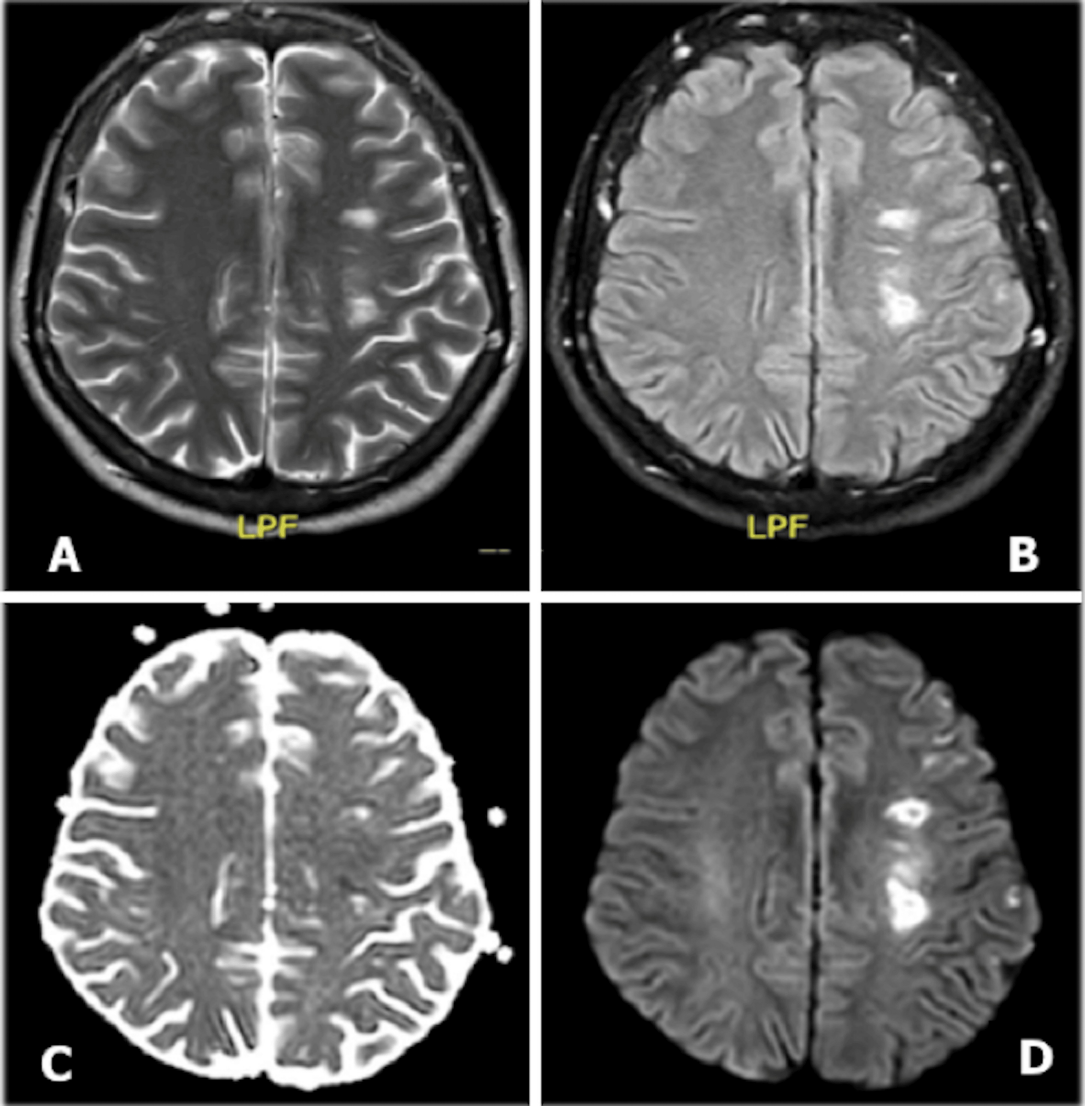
Magnetic resonance imaging with contrast of the brain. Ischemic infarcts in the middle cerebral artery territories, in different stages (acute, subacute, and chronic, with alteration of flow signal in the left carotid artery. (A) T2 sequence. (B) FLAIR sequence. (C) ADC sequence. (D) DWI sequence LPF: local perturbation field; FLAIR: fluid-attenuated inversion recovery; ADC: apparent diffusion coefficient; DWI: diffusion-weighted imaging
